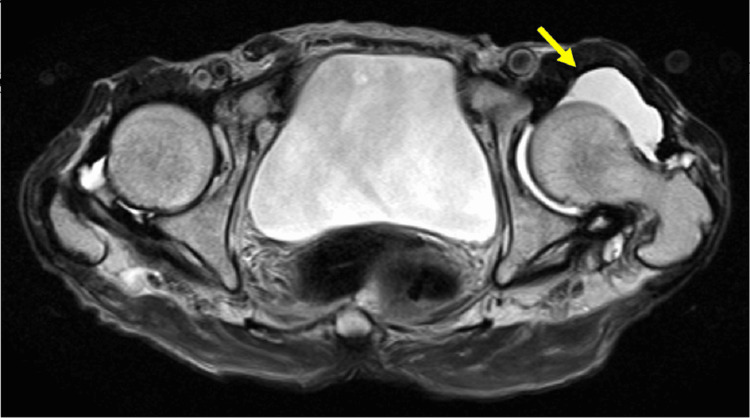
Magnetic resonance imaging (MRI) of the hip joint. T2-weighted MRI showing a small amount of joint effusion, rupture of the joint capsule, marked atrophy of the gluteus muscles, thinning of the iliopsoas tendon, and loosening of the soft tissue on the anterior side of the hip joint.
